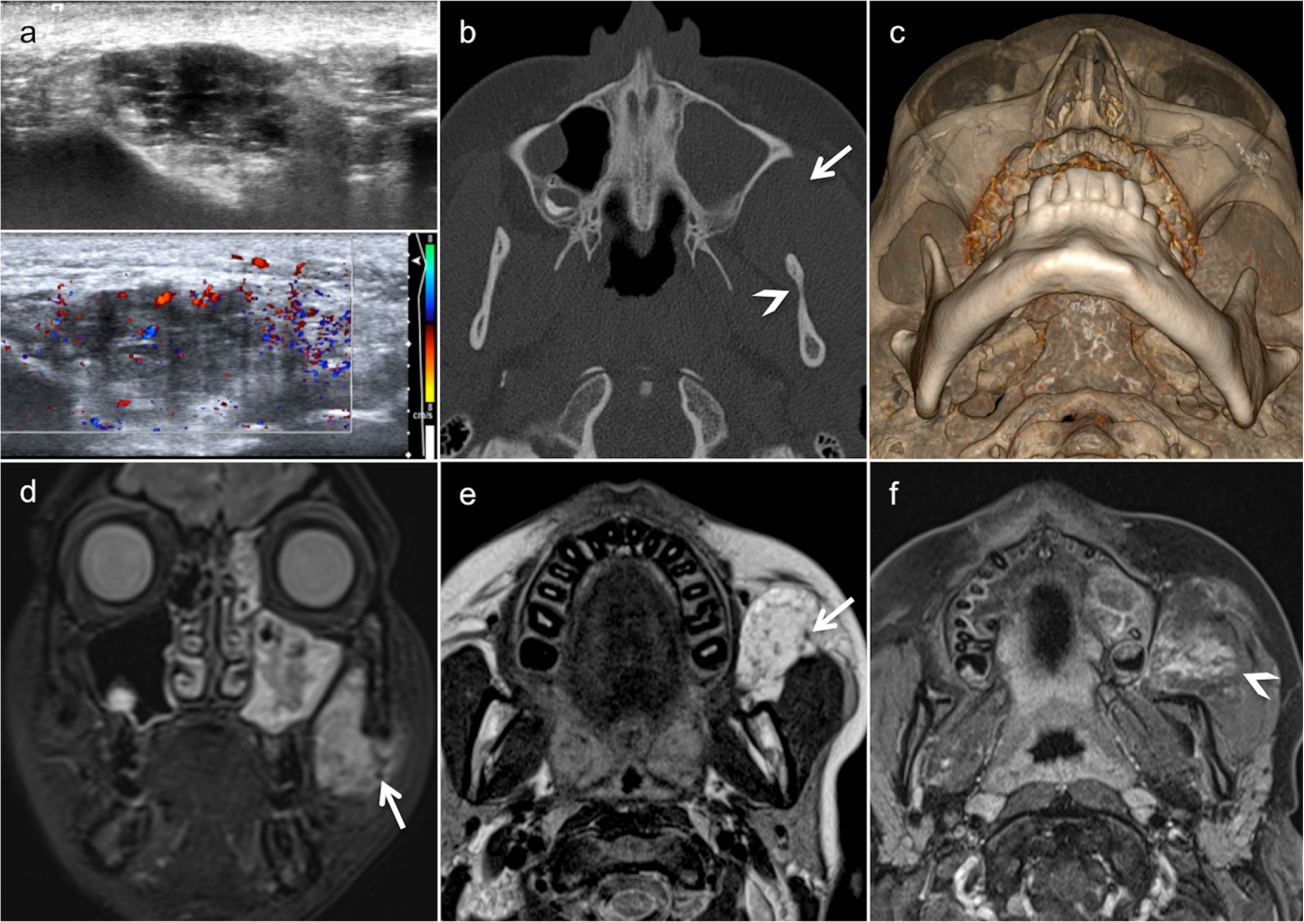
Venous vascular malformation (VVM) in a 12-year-old girl complaining of increasing left cheek swelling causing major facial asymmetry and dental malocclusion. a Grey-scale ultrasound (*upper image part*) and corresponding Doppler US (*lower image part*) show hypoechoic and infiltrative mass with poor vascularisation. b Axial low-dose CT (bone window) shows shortened and remodelled left ascending ramus of the mandible (*arrowhead*) and increased distance (*arrow*) between the left ascending ramus of the mandible and posterior wall of the left maxillary sinus, compatible with a slow-growing lesion. c This increased distance is also clearly seen on the three-dimensional CT VR reconstruction. STIR (d) and T2 (e) images show a hyperintense mass with multiple small phleboliths (small, dark, rounded areas) situated within Bichat’s fat (*arrows*). f Contrast-enhanced axial fat-saturated T1 shows partial, progressive, centripetal lesion enhancement (*arrowhead*) characteristic of a VVM
